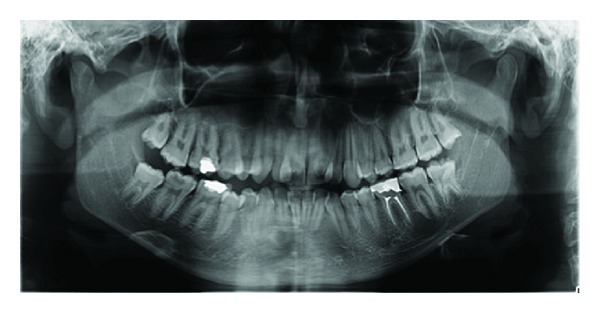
Panoramic radiographic image, which presents permanent dentition with complete teeth number.
